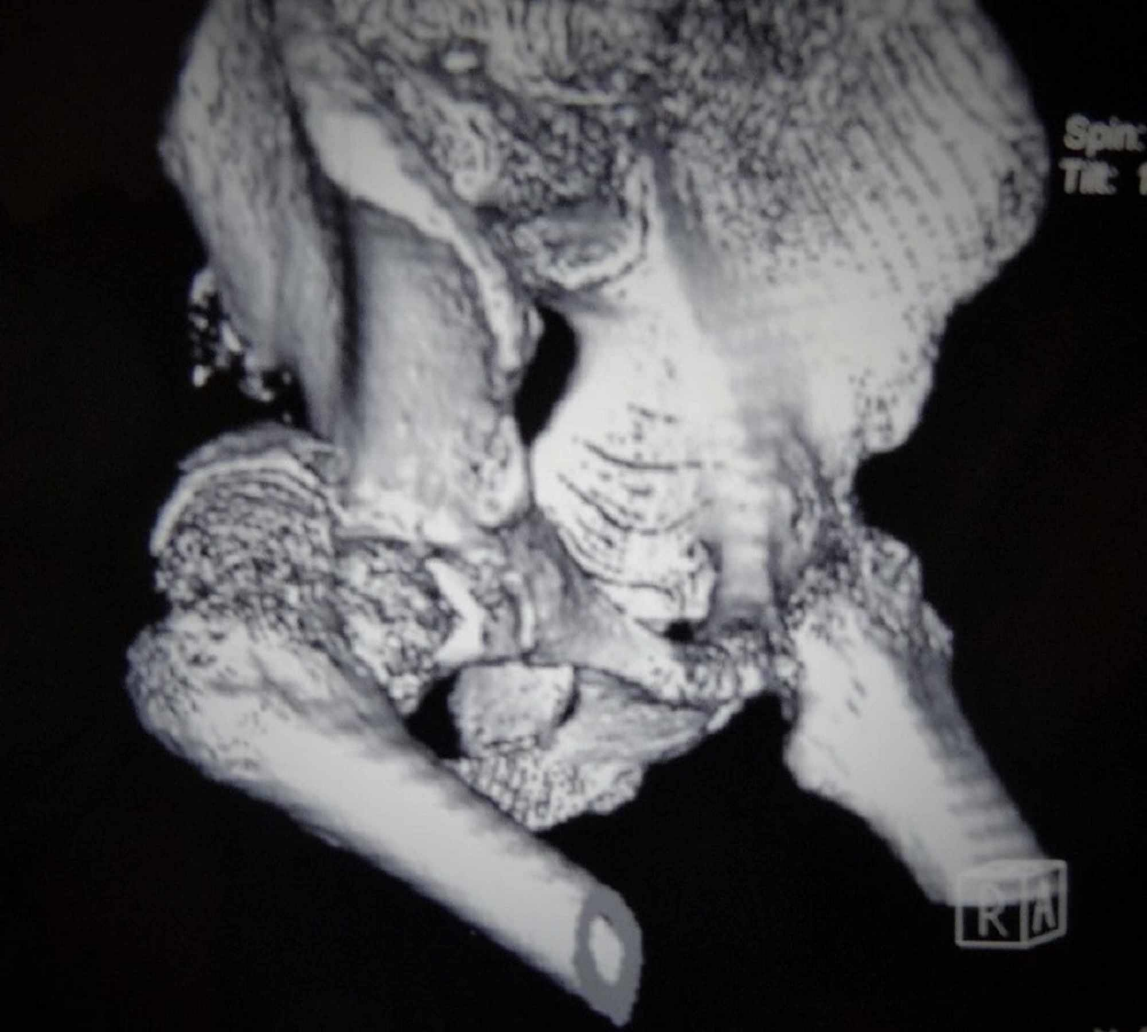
Preoperative CT reconstruction of the same patient
